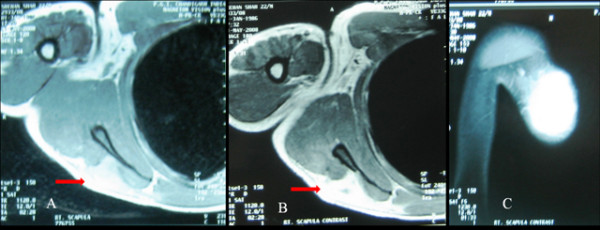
A, B, C: MRI scan in axial and coronal cut sections showing hyperintense image on T1W and T2W sequence, but no significant enhancement noticed in postcontrast images.
